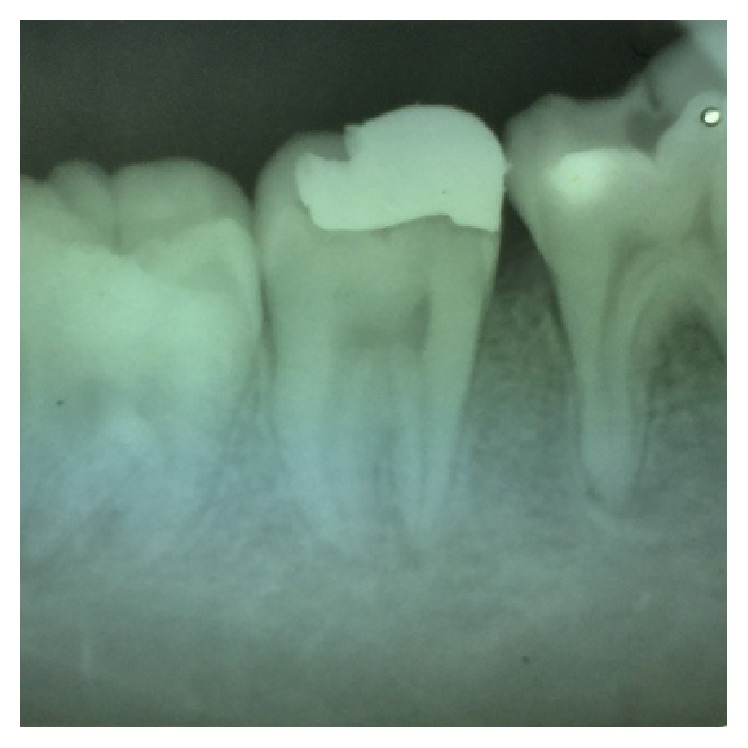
Radiograph showing luted inlay with respect to 37.
